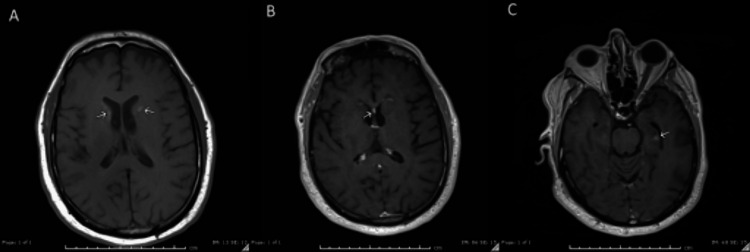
MRI T1 with and without contrast enhancement 24 days after the original MRI (A) T1 without contrast enhancement showing interval increased size of signal abnormalities involving the bilateral caudate nuclei. (B) T1 with contrast enhancement showing interval development of a 5-mm area of enhancement of the septum pellucidum. (C) T1 with contrast enhancement showing interval development of a small focus of patchy ill-defined enhancement within the region of the left hippocampus measuring 6 mm. MRI: magnetic resonance imaging
